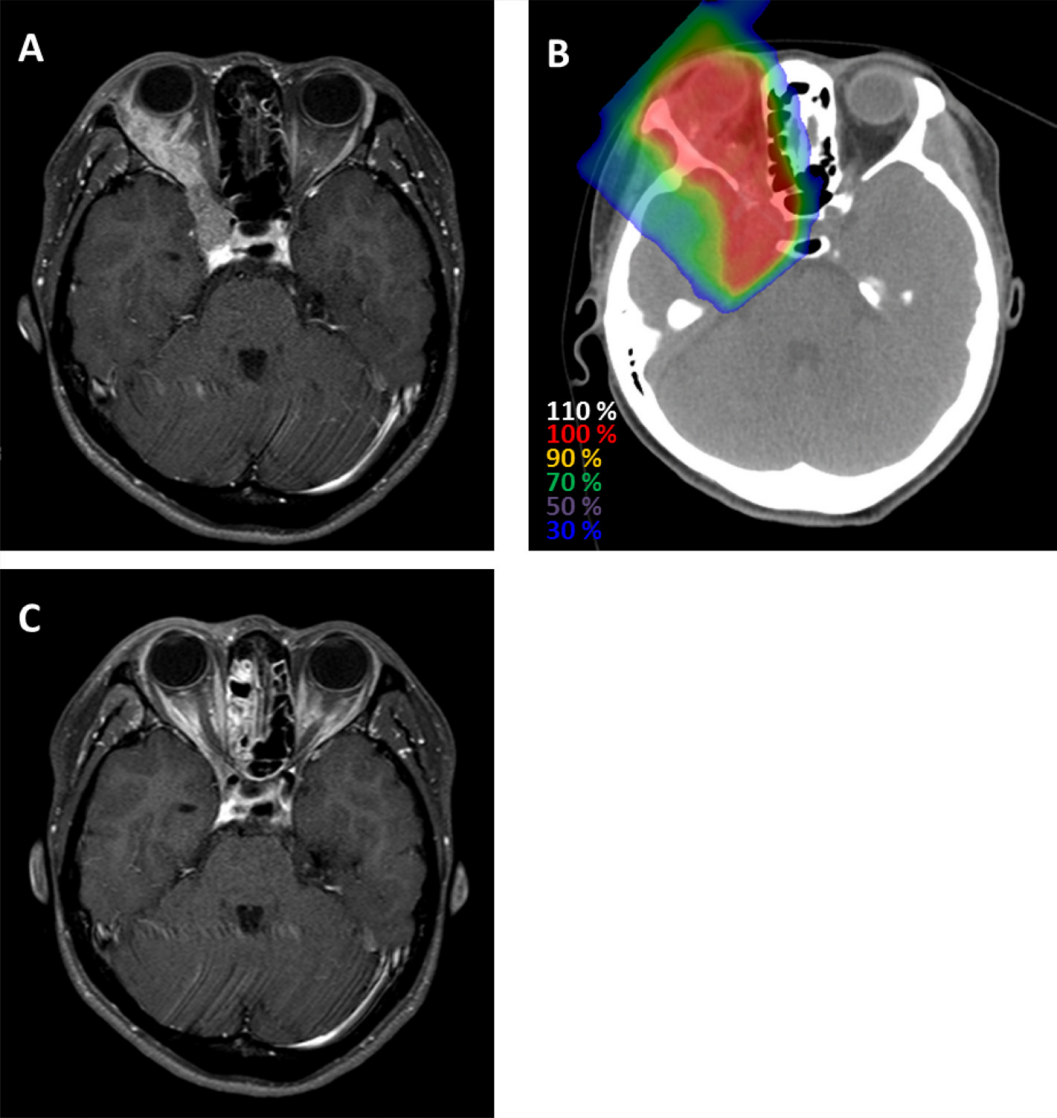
Representative case of a patient with adenoid cystic carcinoma of the right lacrimal gland who was treated with CIRT (A) Magnetic resonance imaging before CIRT reveals a well-enhanced right orbital tumor extending from the right lacrimal gland to the right cavernous sinus (B) Dose distribution. (C) Magnetic resonance imaging 8 months after treatment shows that the tumor had completely disappeared.
